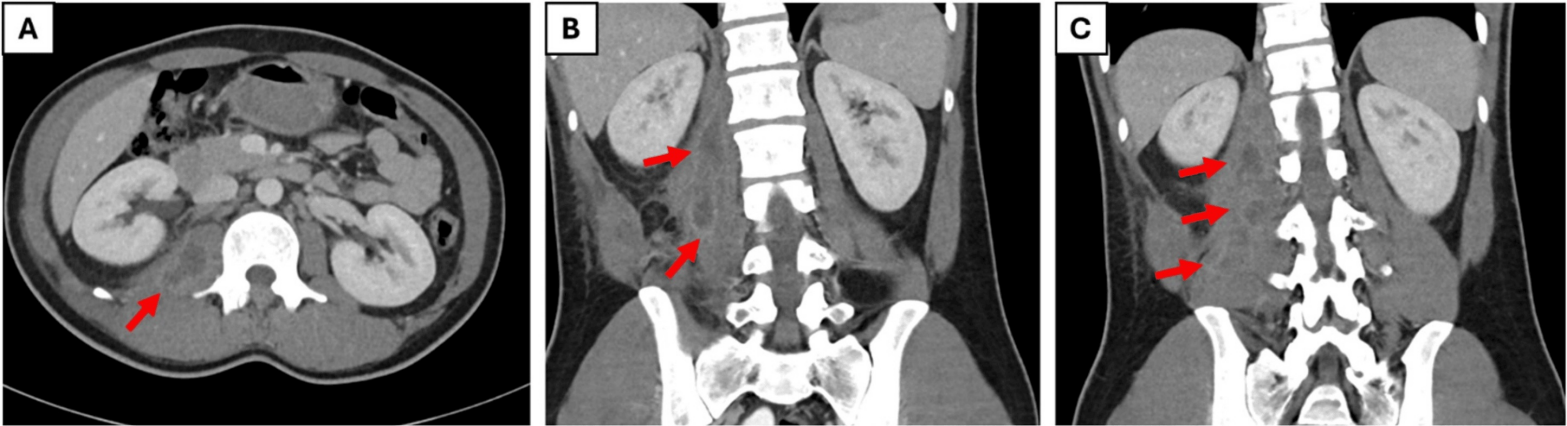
Selected axial (A) and coronal (B, C) contrast-enhanced CT images showing a multiloculated abscess tracking along the entire swollen right psoas muscle into the iliacus, with enhancing septations and surrounding inflammatory changes
